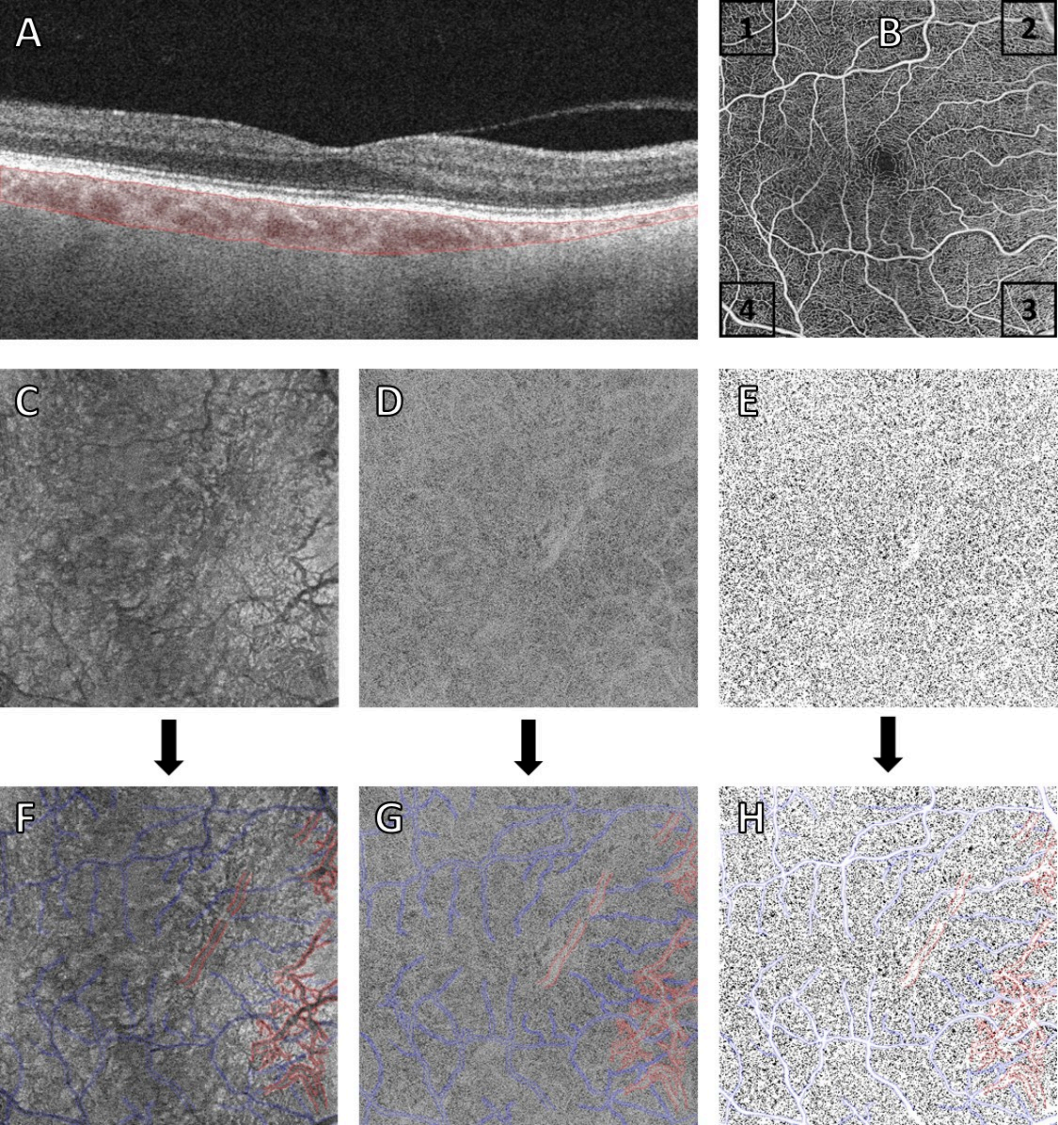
Visualization of choroidal thickness (CT) and en-face choriocapillaris (CC) images throughout different processing steps in 6x6mm scans of a healthy right eye. (A) CT (marked in red) in a B-scan showing a thickness decrease towards the nasal half of the image. (B) Layout of the four squares in a right eye. (C-E) en-face images of the CC-slab, showing the structural information scan (C), the signal compensated CC scan (D) and the binarized version of the signal compensated CC scan (E). The bottom set of images (F,G,H) shows the projection of the superficial plexus, marked in blue and large choroidal vessels (LCV) marked in red on the images above. Additionally, in the last image (H) the superficial plexus was masked for better visualization.
